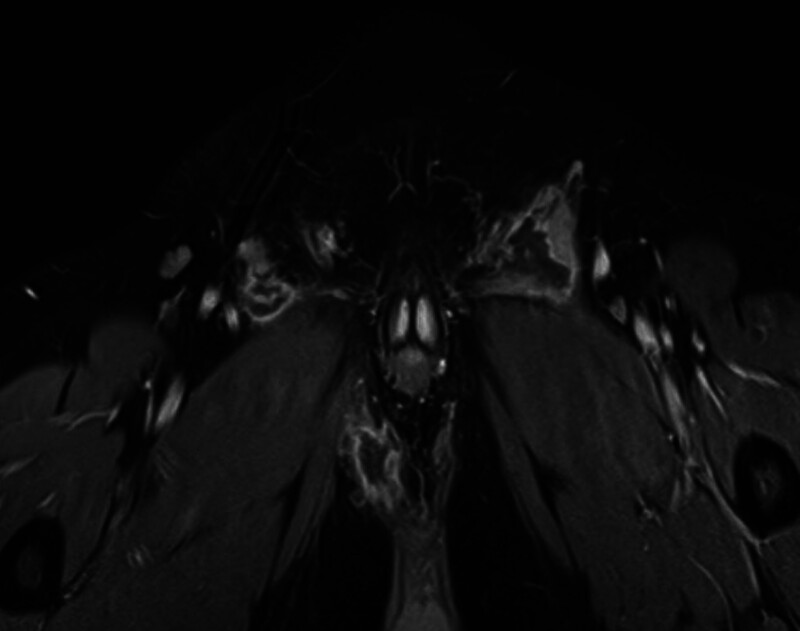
MRI showed diffuse multiloculate large deep-seated anorectal sepsis. MRI = magnetic resonance imaging.
